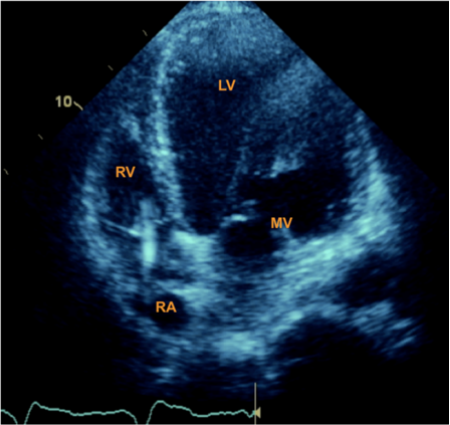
Grossly dilated left ventricle with biventricular pacing wire present in right heart in the apical four-chamber view. *LV* left ventricle, *MV* mitral valve, *RA* right atrium, *RV* right ventricle
